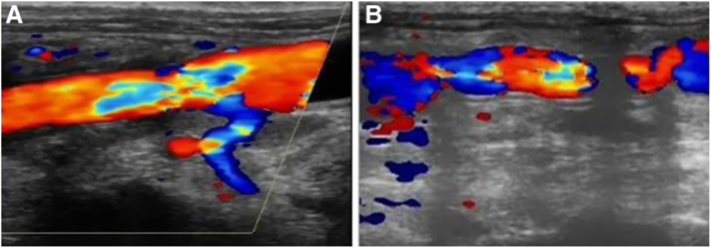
Color Doppler ultrasound images taken 12 weeks after the surgery. (A) A PU graft, (B) A PTFE graft.
